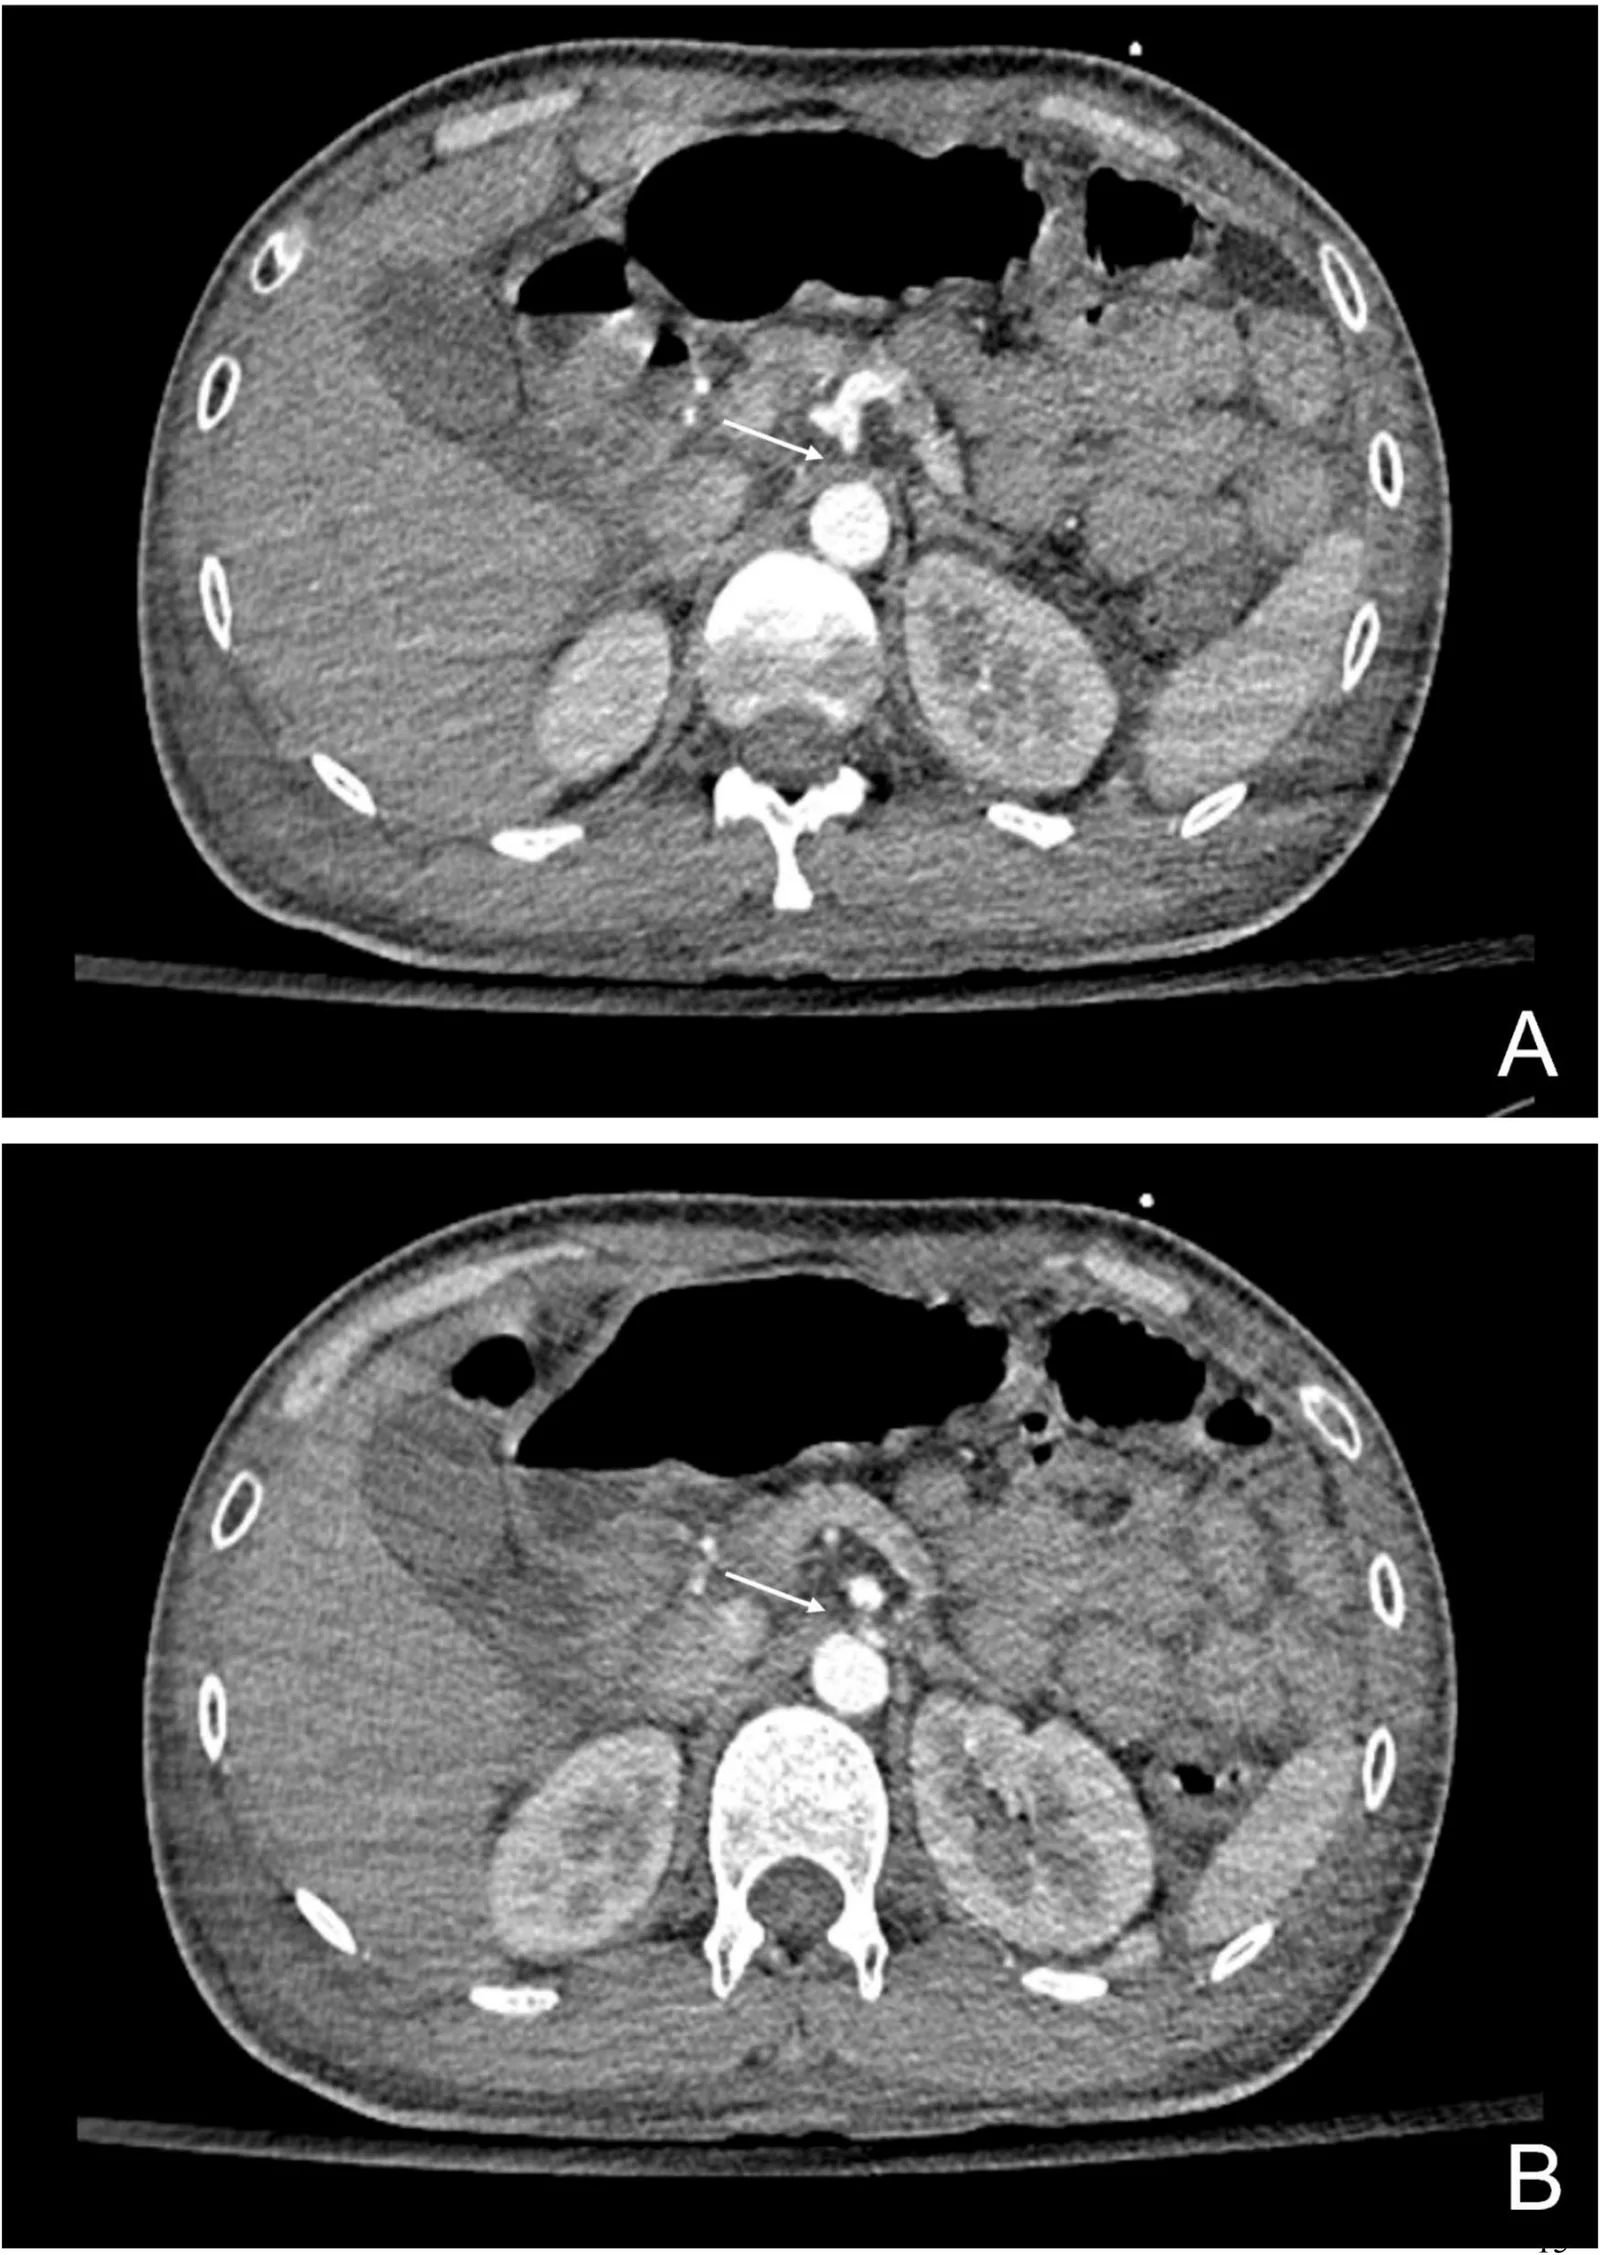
(A, B) Results of chest enhanced CT scan. Review of the chest enhanced CT showed that the proximal celiac trunk was markedly stenosed at the time of admission, but this finding was not reported.
